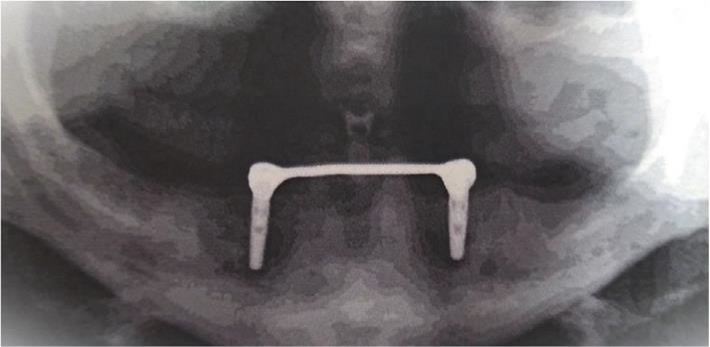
X-ray showing a customized cobalt–chromium metal bar and clip installed for the implants.
